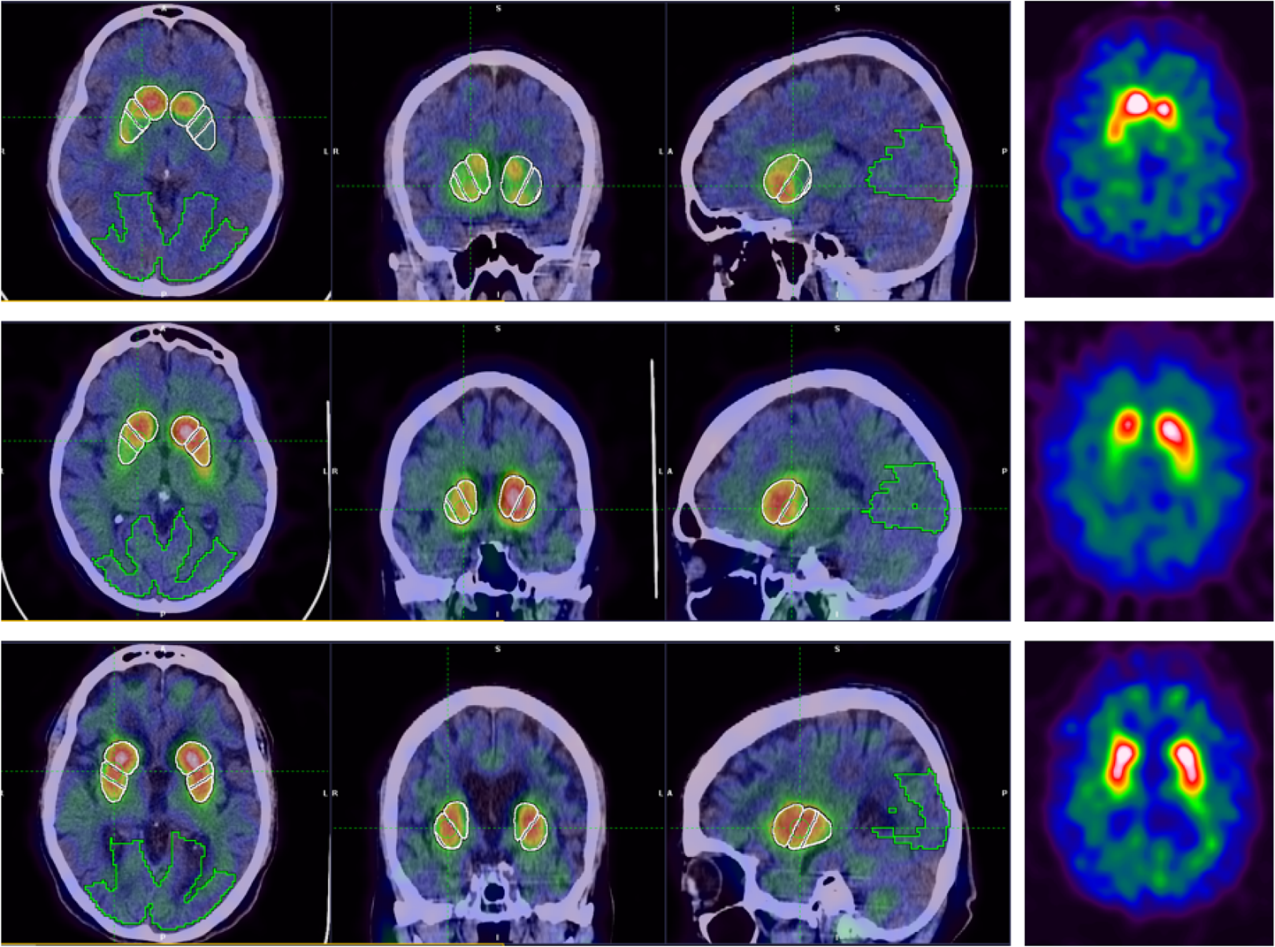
Examples of three test cases used to validate the generated databases: two positive (first and second rows) and one negative (third row) case. Each row from left to right, axial, sagittal, and coronal fused view of the the patient’s SPECT and CT images showing the striatal and occipital VOIs, and the corresponding slab view images (Buchert et al. 2016b) generated within the *syngo*.via® software and used for visual assessments
